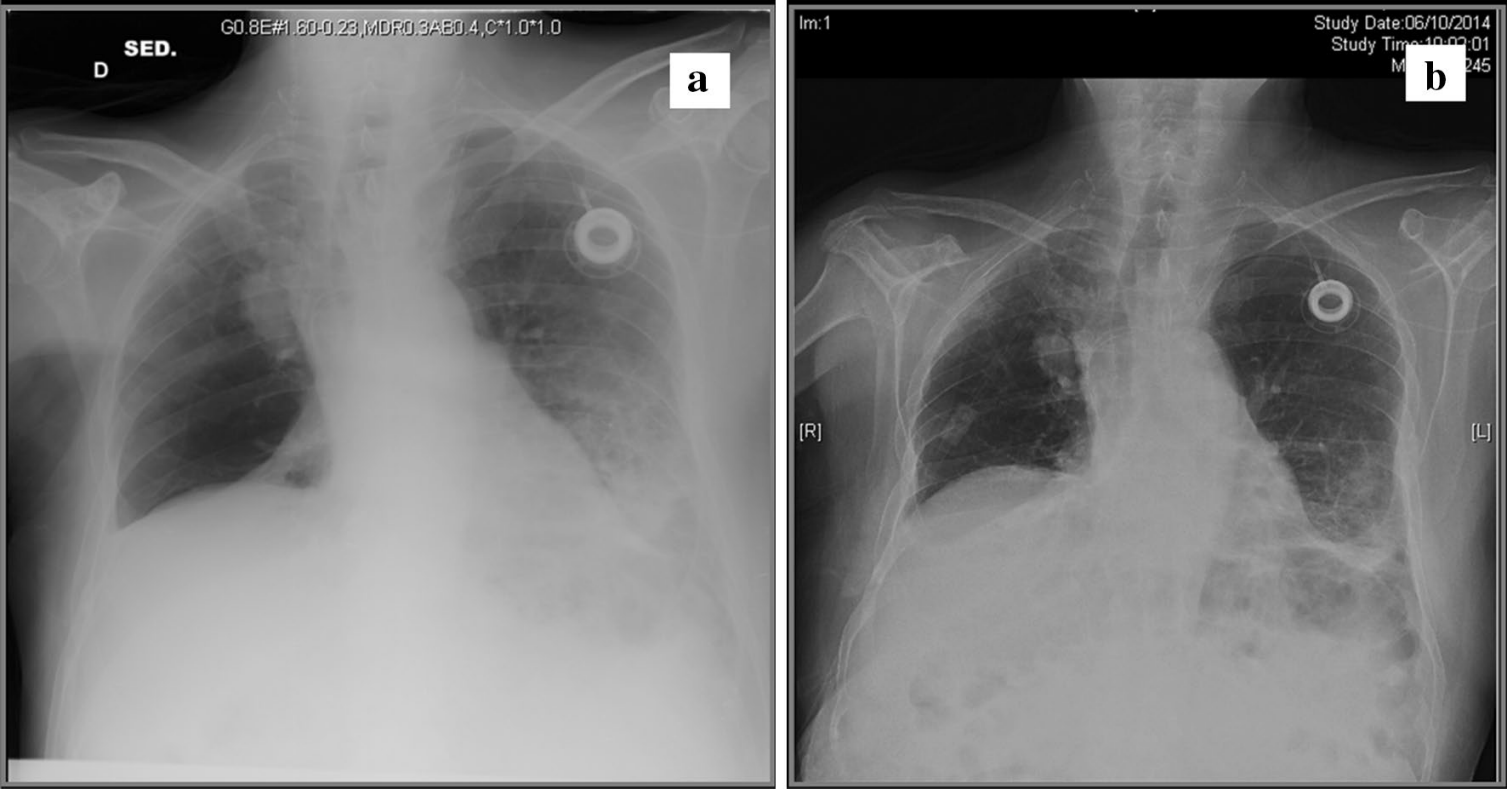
a At the start of the fifth cycle dispnea was evident. A chest radiography was done which was strongly suggestive of pneumonitis then we interrupted treatment with everolimus exemestane and we started him on systemic therapy steroid, bronchodilators and supplemental oxygen. b A new chest radiography was taken 10 days after cessation of treatment and highlights an improvement in the pneumonitis
